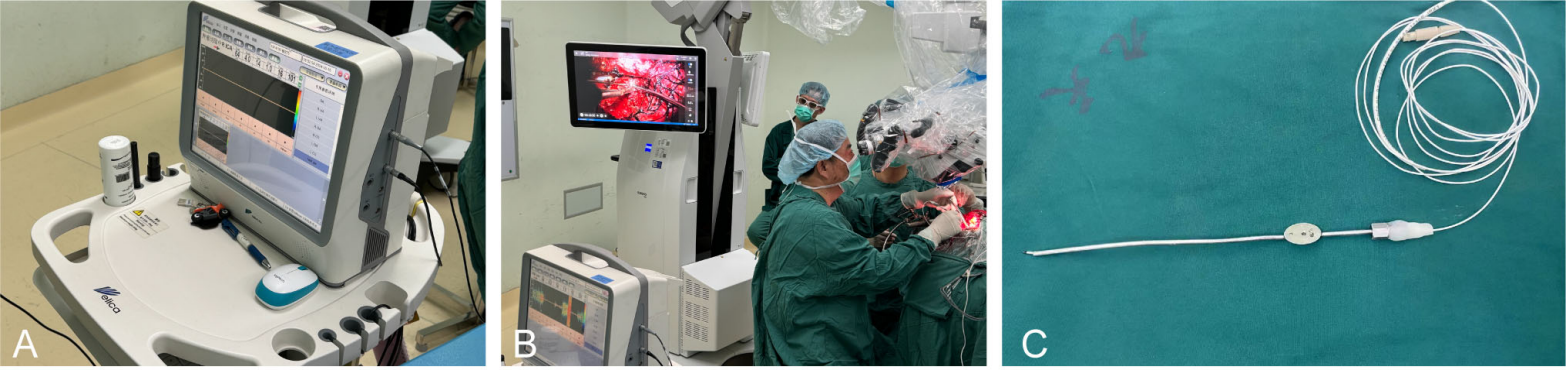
Intraoperative microvascular Doppler used in the surgery. (A) German transcranial Doppler device. (B) Use MVD for real-time monitoring during surgery. (C) The ultrasound probe.
